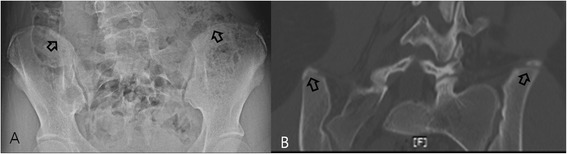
Pseudo-fusion. (A) Plain radiography of a 15-year-old girl; a sclerotic line was visible on the posterior iliac crest suggesting fusion of the iliac apophysis, and the patient was graded as Original Risser stage 5 and French Risser stage 4. (B) A coronal 3D reconstruction view; there was no definite fusion of the apophysis , and the patient was graded as Original Risser stage 4 and French Risser stage 3. Estimating the initiation of pseudo-fusion is difficult on plain radiography.
